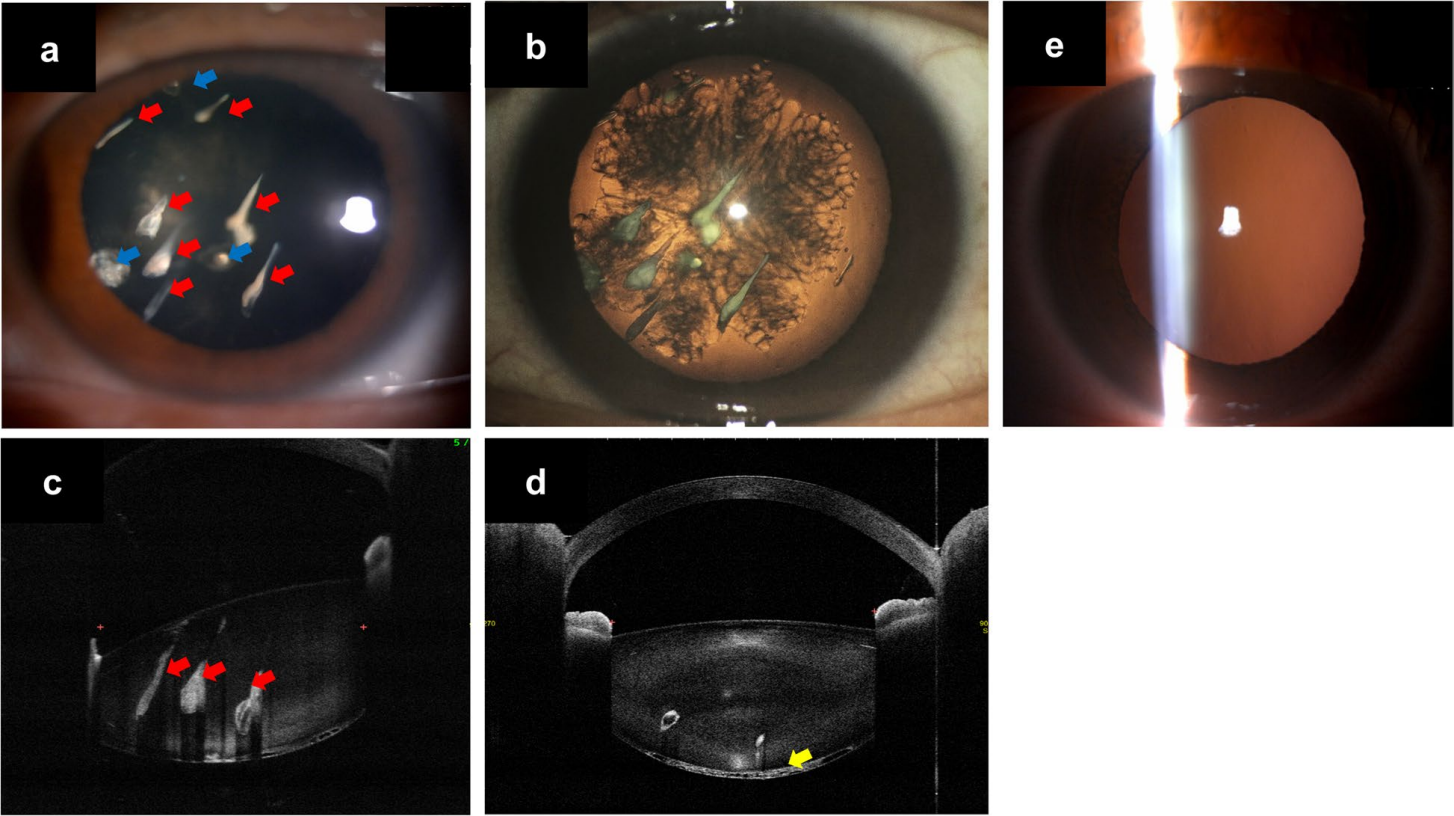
Eyes, seven days after the intense focused ultrasound treatment. Several drop-like opacifications and rosette-like posterior subcapsular cataract (PSC) can be observed in the patient’s left eye, which were documented as acute cataract by slit-lamp photography. a Seven drop-like dense lens opacities were aligned and obliquely localized from the center of the lens to the perinucleus region (red arrows). Three lens sense opacities extended vertically to the posterior capsule (blue arrows) by slit-lamp photography. b Retro-illumination image of the patient’s left eye by a Casey Eye Institute camera system [4]. Rosette-shape PSC was observed. c d Anterior optical coherence tomography showing drop-like opacity (red arrows) in the patient’s lens (c) and PSC (yellow arrow) (d); the patient’s posterior capsule did not appear to be ruptured. e No lens opacity was observed in the patient’s right eye
